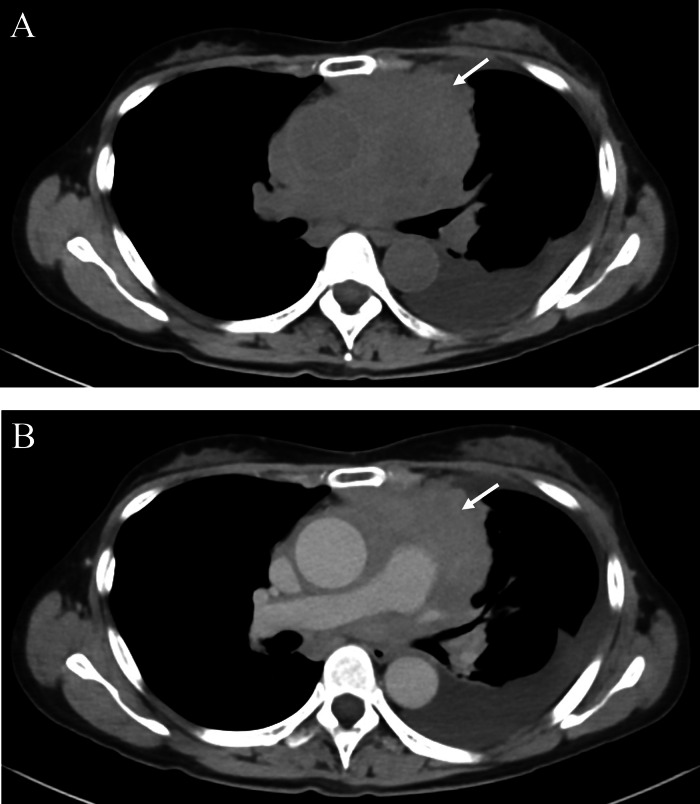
Axial images of plain CT-scan (A), contrast-enhanced CT-scan (B) in the first case. Axial CT images showing poorly defined irregular soft tissue mass (arrow) from the anterior to the middle mediastinum. The mass exhibits homogeneous enhancement (arrow in B). Left pleural effusion is observed.
